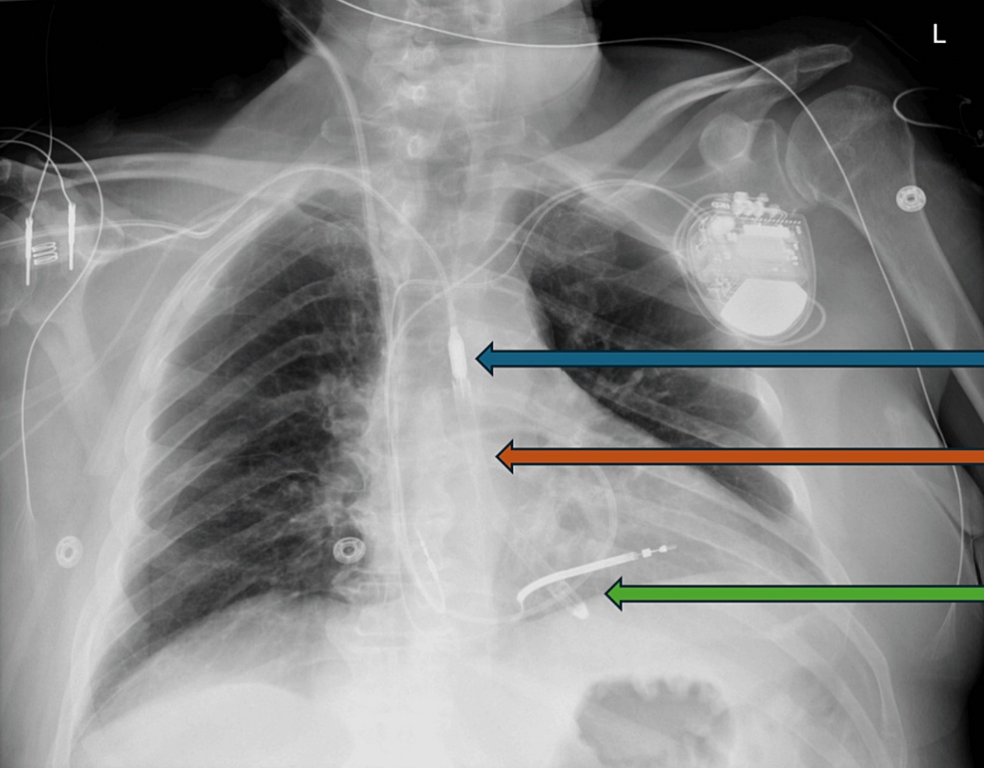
Chest X-ray was obtained to confirm appropriate Impella placement across the aortic valve and into the left ventricle. The Impella outflow (blue arrow), approximate position of the aortic valve (orange arrow), and left ventricle (green arrow) can be observed. The Impella device is directed towards the apex and 5 centimeters deep into the left ventricle from the aortic valve.
